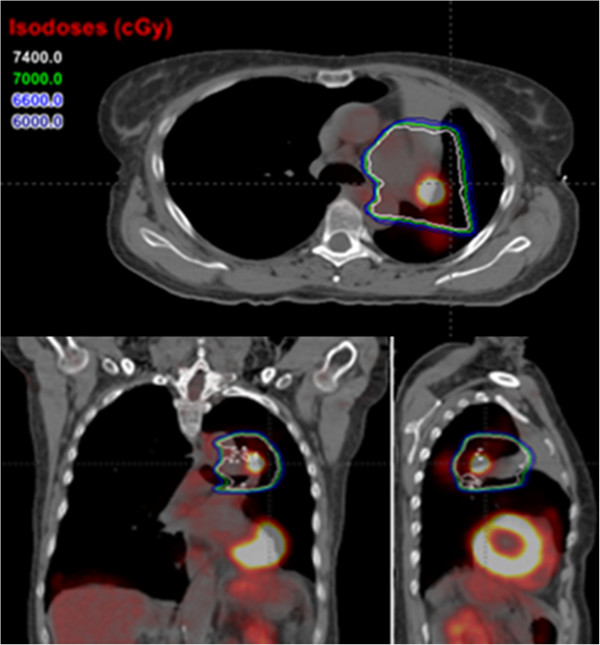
Axial, coronal, and sagittal views of fused PET/CT scans showing a local recurrence that occurred within both the 74 Gy(RBE) (white) isodose lines on the worst-case dose distribution.
